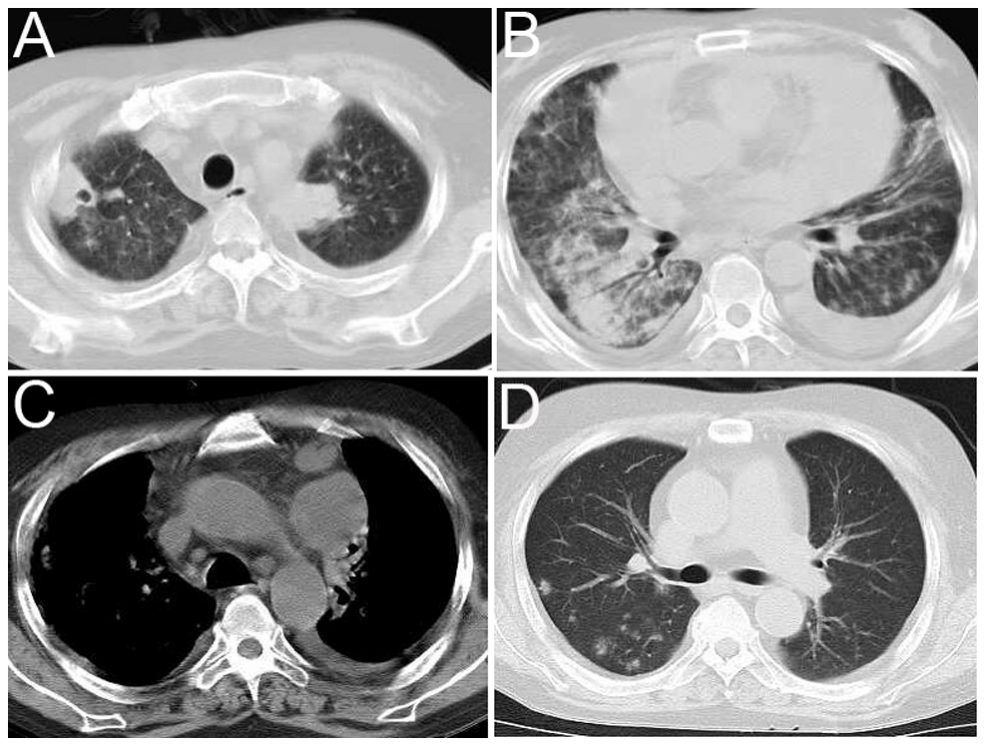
A 45-year-old man on immunosuppressive therapy after renal transplantation was infected with *Nocardia asteroides*. A–C, Axial CT images reveal multiple patterns of disease and lymphadenopathy; D, An axial CT image reveals small pulmonary nodules with relapsed infection two years after the initial diagnosis.
